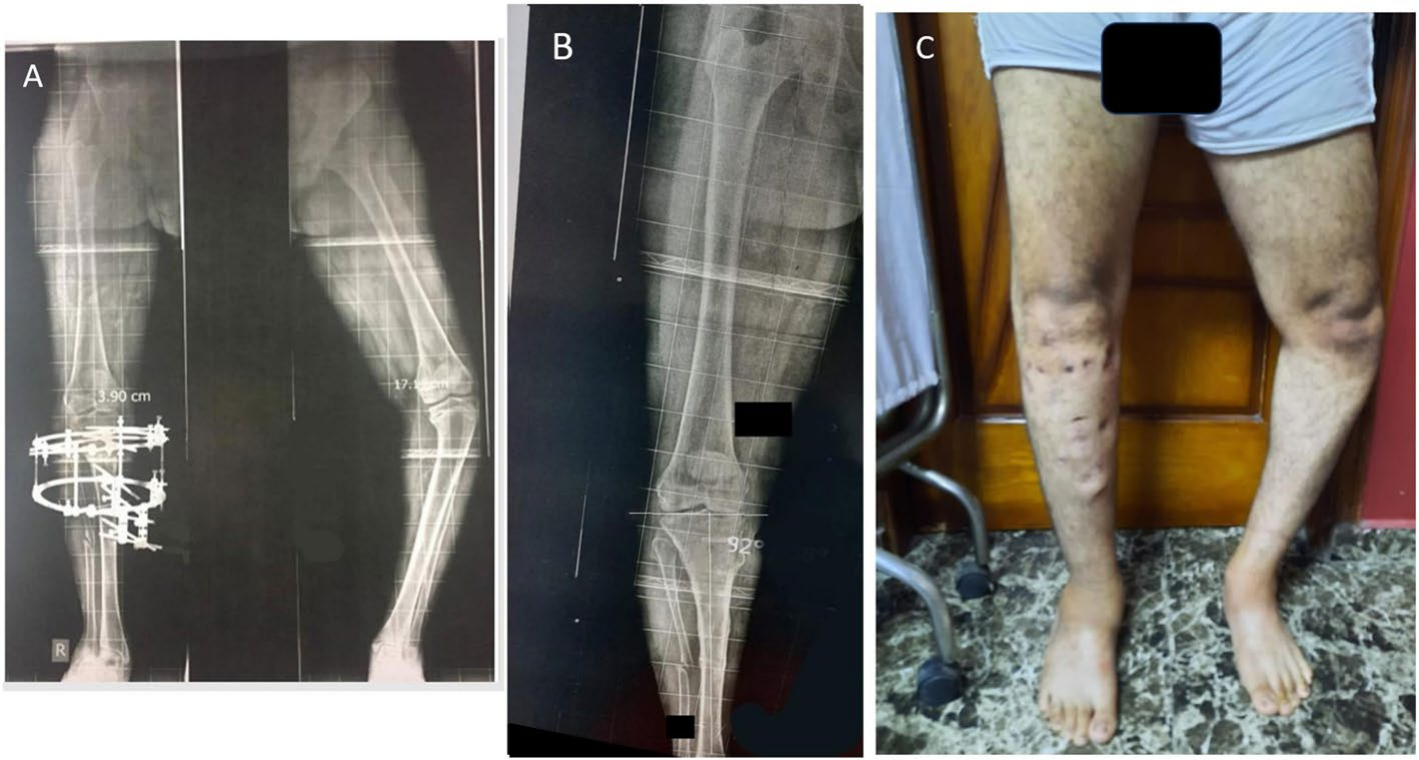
Post-operative scanogram 3 months follow-up before Ilizarov frame removal with normalization of the mechanical axis (A). Radiograph (fully united) and clinical appearance after Ilizarov frame removal 3.5 months of the previously prescribed patient (B, C)
